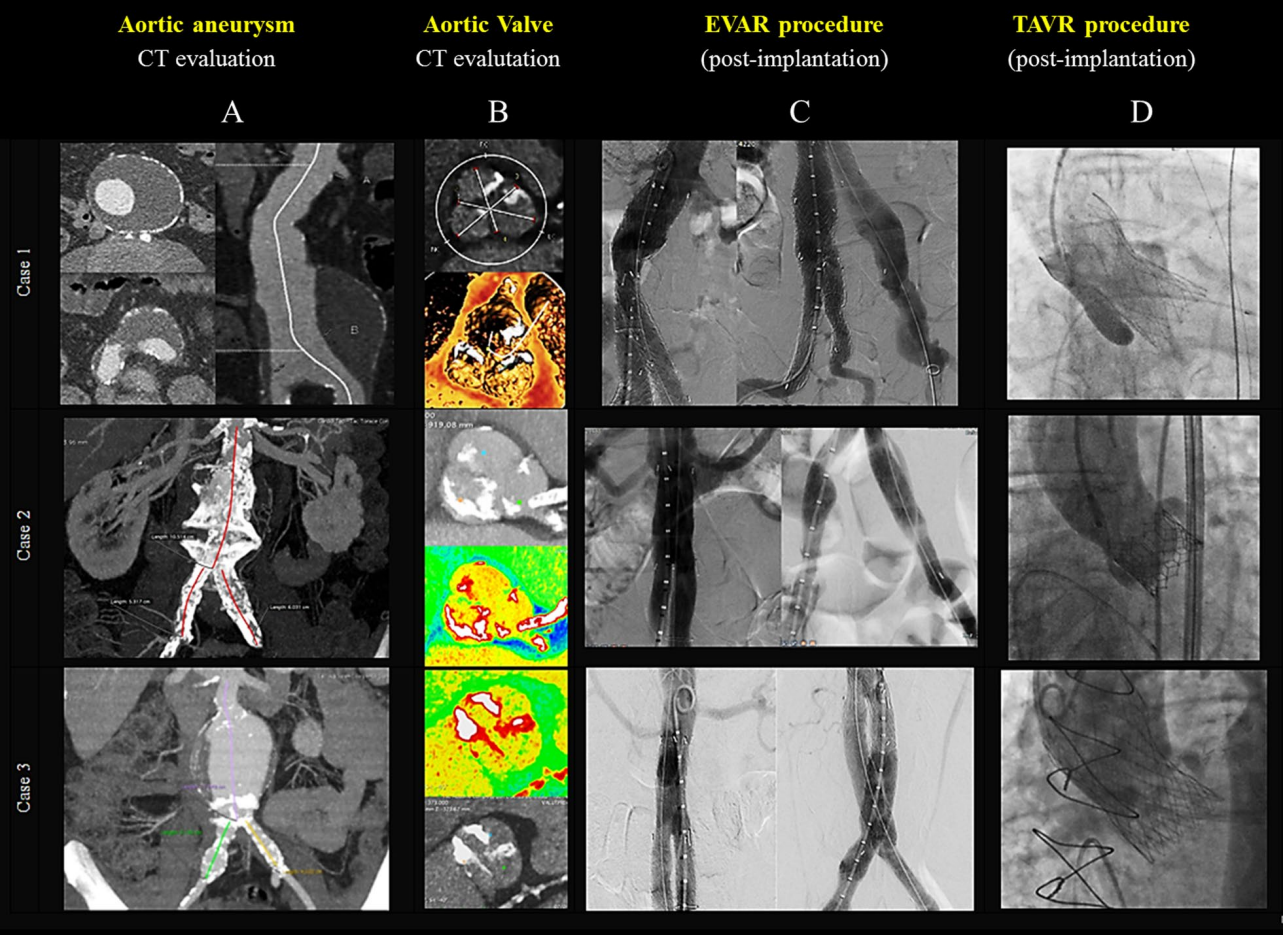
Cases 1–3 pre-procedural images of computed tomography (CT) angiography with tridimensional reconstruction of abdominal aortic aneurysm (panel A) and anatomy of the aortic valve (panel B). Post-procedural EVAR (panel C) and TAVR (panel D) results
